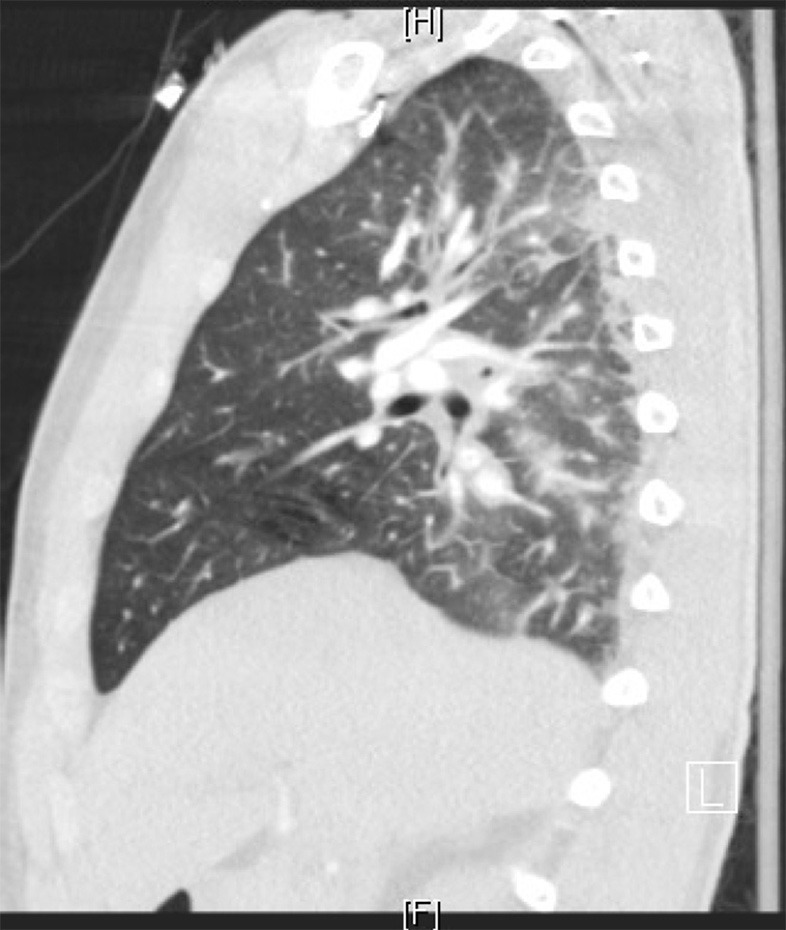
High resolution CT sagittal view of left lung and right heart border. It showed intralobular septal thickening with ground glass opacities consistent with pulmonary edema. Bilateral lower lobe bronchial wall thickening with bronchial debris and consolidative opacities were present. It did not show evidence of pulmonary embolus.
